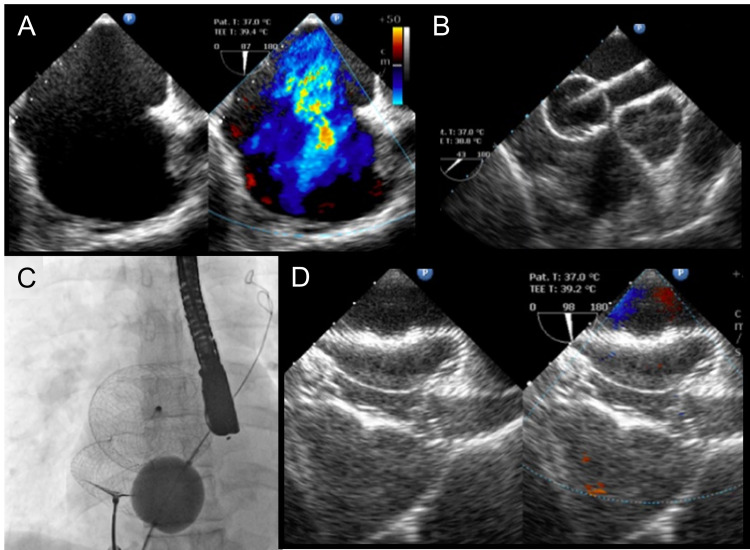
(a) Transesophageal echocardiography (TEE) with color Doppler showing a very large atrial septal defect with left-to-right shunt and thin and floppy posterior inferior margin. (b) TEE showing Equalizer balloon across the defect. (c) Fluoroscopic image showing balloon-assisted device closure of the defect. (d) TEE with color Doppler showing good device occlusion.
